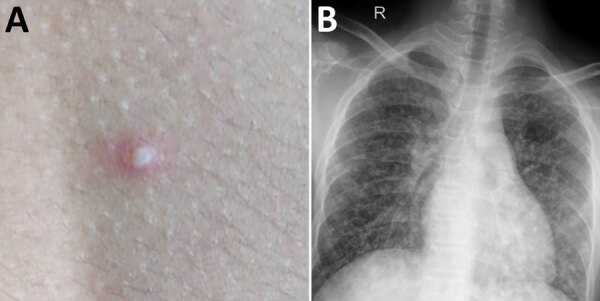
Images of 15-year-old boy (patient 1) with melioidosis, Kerala, India, 2019. A) Vesicular lesion on trunk. B) Chest radiograph showing diffuse heterogeneous opacities suggestive of acute respiratory distress syndrome.
